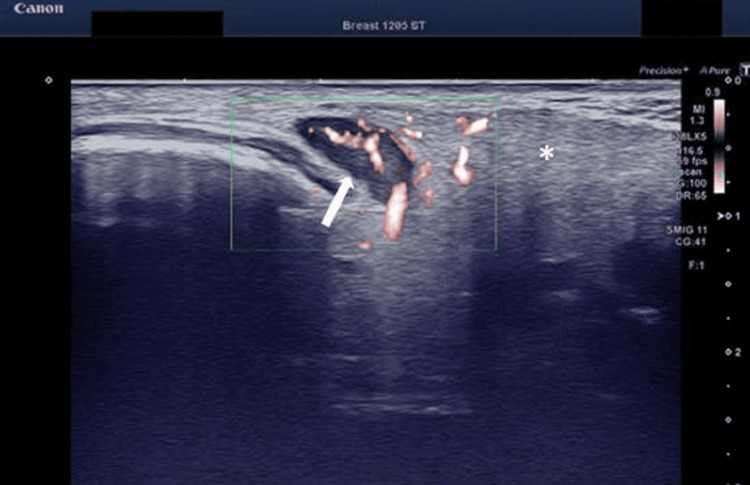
Color Doppler image demonstrates a swollen lymph node (white arrow) with hyperemia and infiltration (asterisk) of the surrounding soft tissue in the posterior cervical region, indicative of lymphadenopathy
